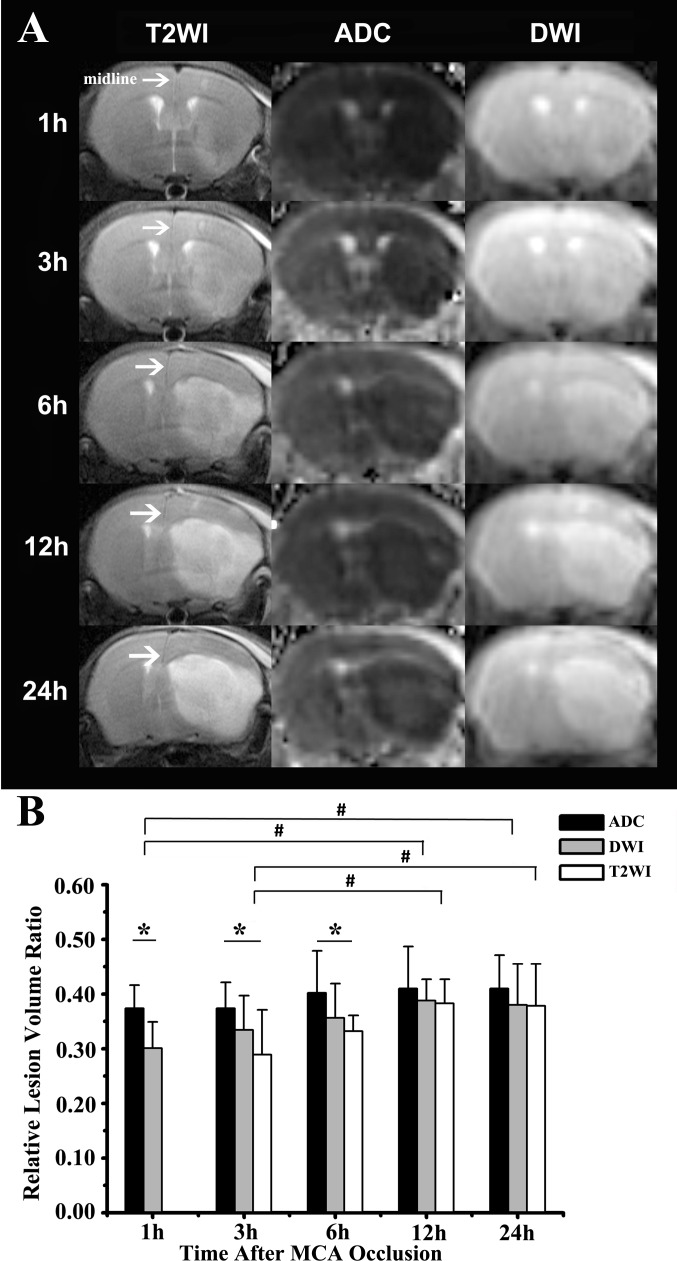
The evolution of the infarct volume on MRI. A) The brain lesions were detected by T2WI, ADC map and DWI at different time points. The curving midlines (white arrows) indicate a severe brain edema. B) The ADC map showed relatively larger lesion volumes at 1 hour (p*<0.05) versus DWI, 3 hours and 6 hours (p*<0.05), and versus T2WI. The relative lesion volumes on DWI and T2WI reached a maximum and matched well with those on ADC map at 12 hours (*p*>0.05), On T2WI, the lesion volumes at 12 and 24 hours were larger (#*p*<0.05) versus that at 3 hours; while on DWI the volumes at 12 and 24 hours were larger (#*p*<0.05) versus that at 1 hour.
